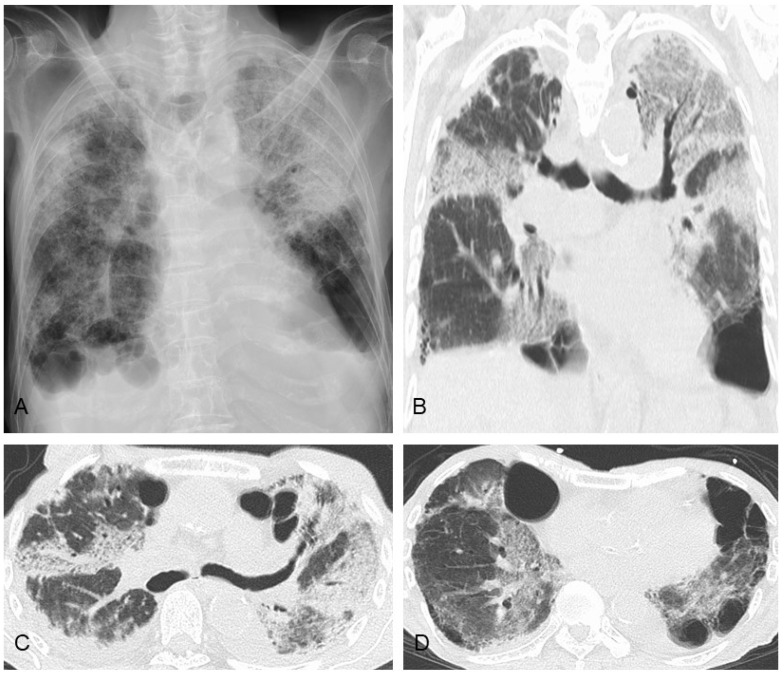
A patient with pleuroparenchymal fibroelastosis in whom acute exacerbation developed. (A) Chest X-ray. Bilateral consolidation with traction bronchiectasis and enlarged ground-glass opacities are shown on chest computed tomography (B–D). A small bilateral pleural effusion was also detected.
